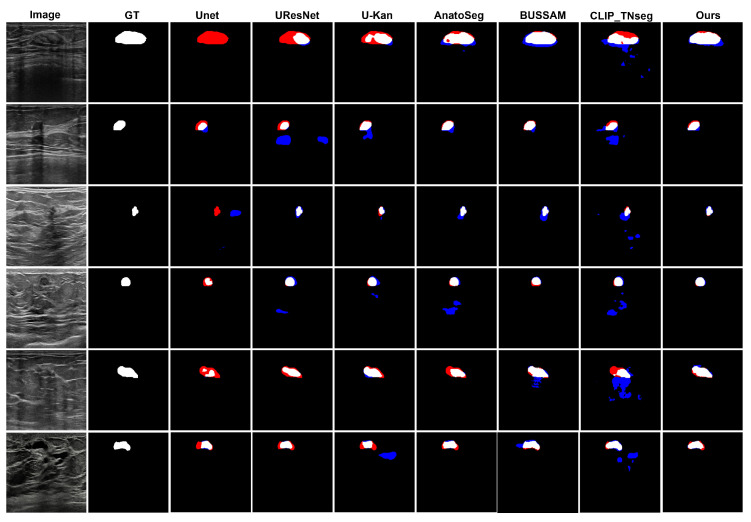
Qualitative comparison of breast ultrasound segmentations. False positives (FPs) are shown in blue and false negatives (FNs) in red.
